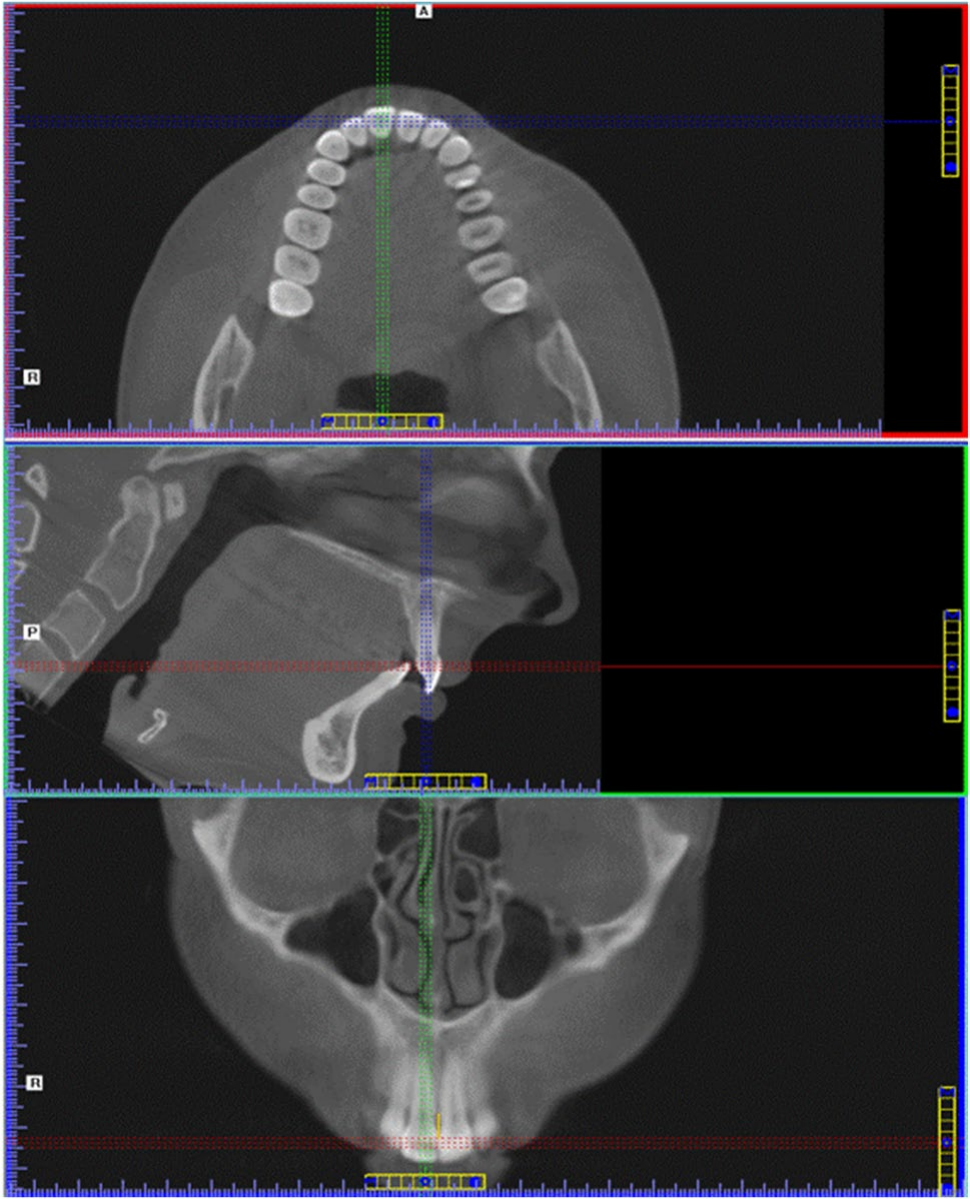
CP-B measurement on CBCT images. A (upper): the crown of tooth 11 was centered in the axial plane. B (middle): the pulp of tooth 11 was centered in the sagittal plane. C (lower): the yellow line gives the CP-B distance in the coronal plane
